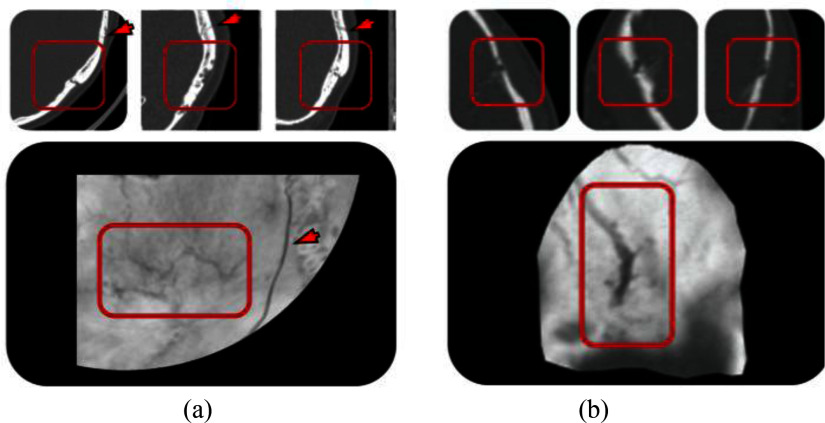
Comparison of emissary veins versus fracture in (a) disk harmonic mapping for Patient 248 and (b) ADR [25]. Top: Multi-planar reformats (axial, sagittal, coronal) CT slices magnified views of the abnormality regions. Bottom: corresponding flattened views. The rectangles indicate the emissary veins. Arrows indicates the fracture location. The fracture in (a) was missed by 2 out of 3 radiologists.
